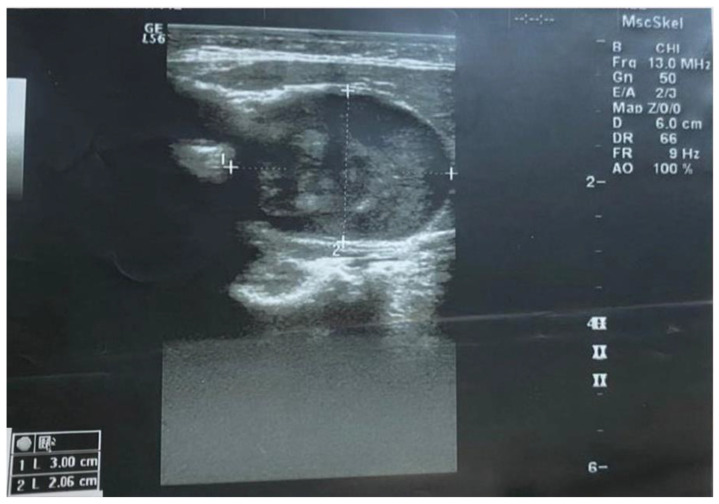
Ultrasound of the neck mass showing a thick-walled cyst with clear contents located on the left side, mesial to the sternocleidomastoid muscle. No flow was observed in the cyst or in the wall of the cyst upon ultrasound Doppler examination.
